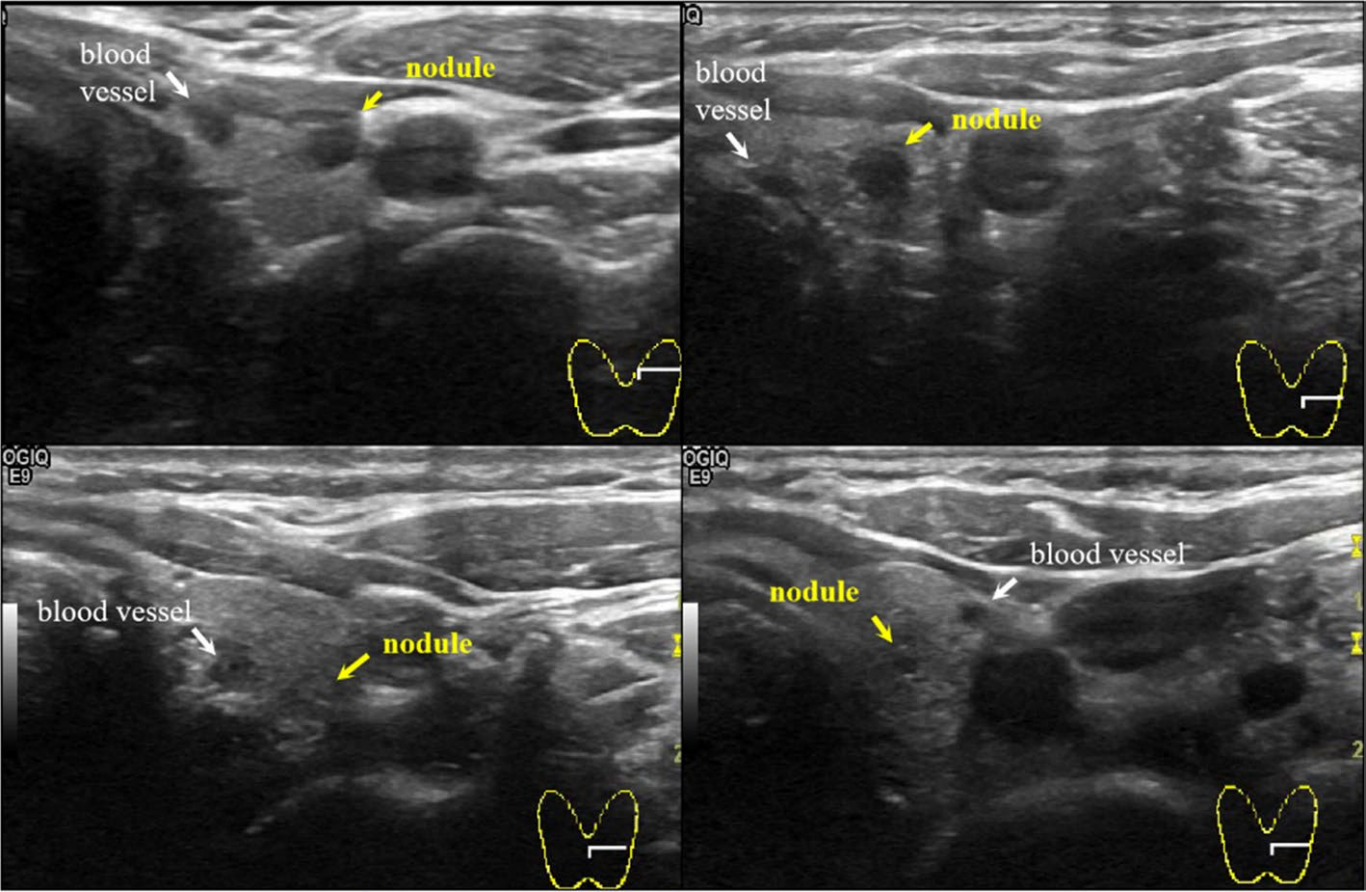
In thyroid ultrasound images, it is easy to get confused between vessels and nodules as they appear extremely similar
